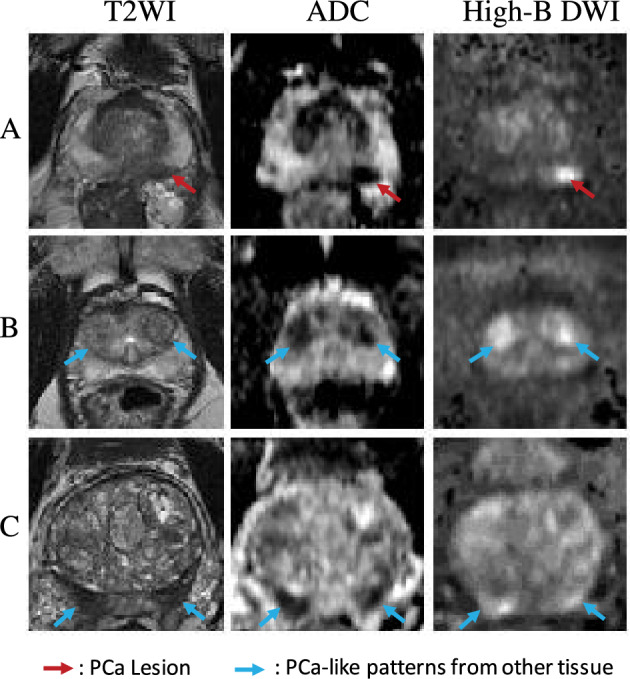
Examples of a PCa lesion and PCa-like patterns from other prostate tissues, like the BPH and the CZ, that can cause FP predictions from three mpMRI scans (A, B, and C). From the left to the right, the first column shows the T2WI images, the second column shows the ADC images, and the third column shows the high-B DWI images. Red arrows point to a PCa lesion, and blue arrows point to the PCa-like patterns in BPH (B) and CZ (C).
